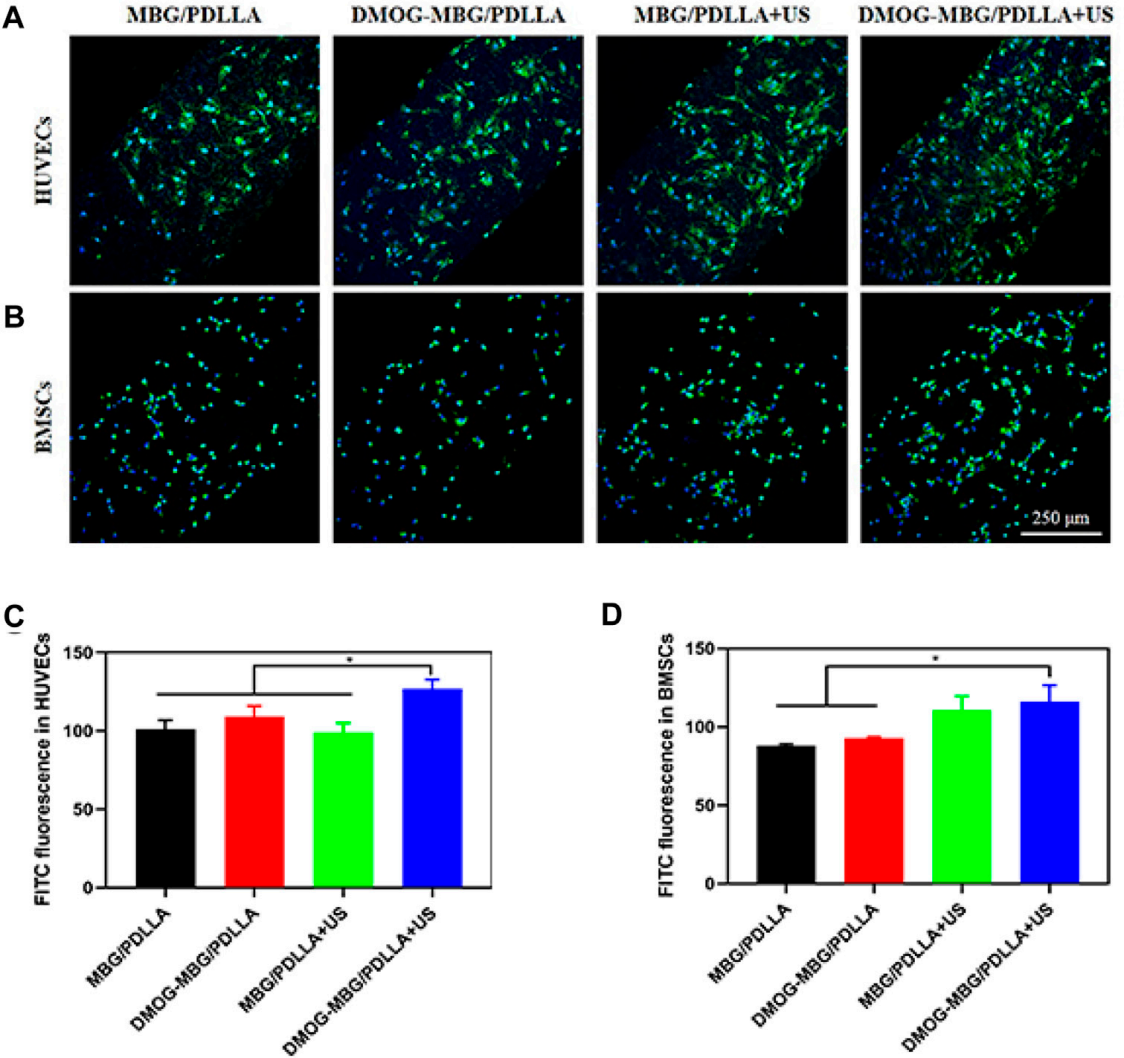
The adhesion of (A, C) HUVECs and (B, D) BMSCs on the MBG/PDLLA and DMOG-MBG/PDLLA scaffolds with and without ultrasound stimulation.
